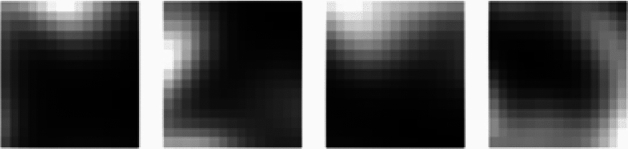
Patches obtained from MRI images.
